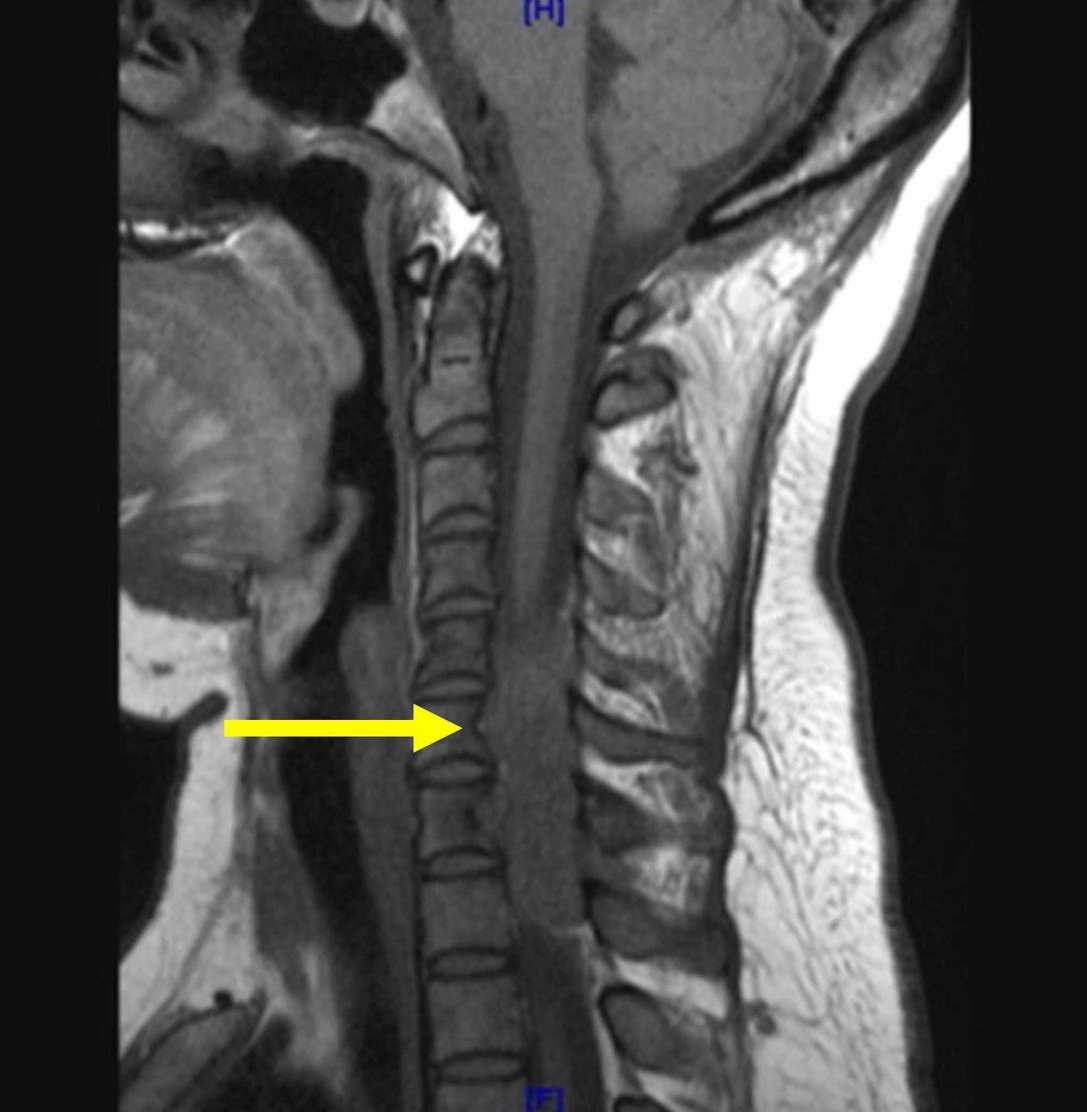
MRI cervical spine. Left epidural enhancing mass measuring 1.4 x 3.5 x 4.6 cm causing severe spinal cord compression from C6-T1, with infiltrative spread into the paravertebral soft tissues.
